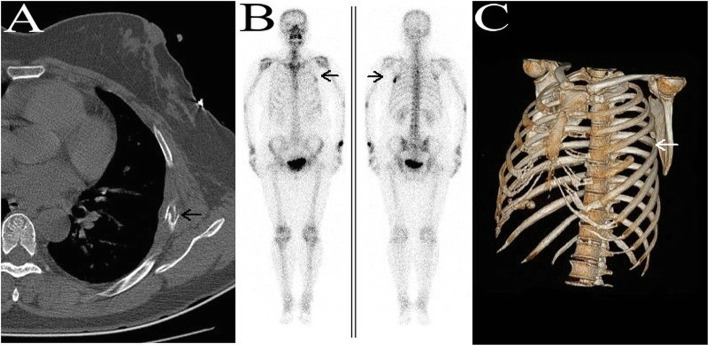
a. Preoperative chest CT suggests pathological fracture. b. Bone scanning suggests increased local metabolism of the lesion. c. Postoperative CT of chest wall reconstruction
